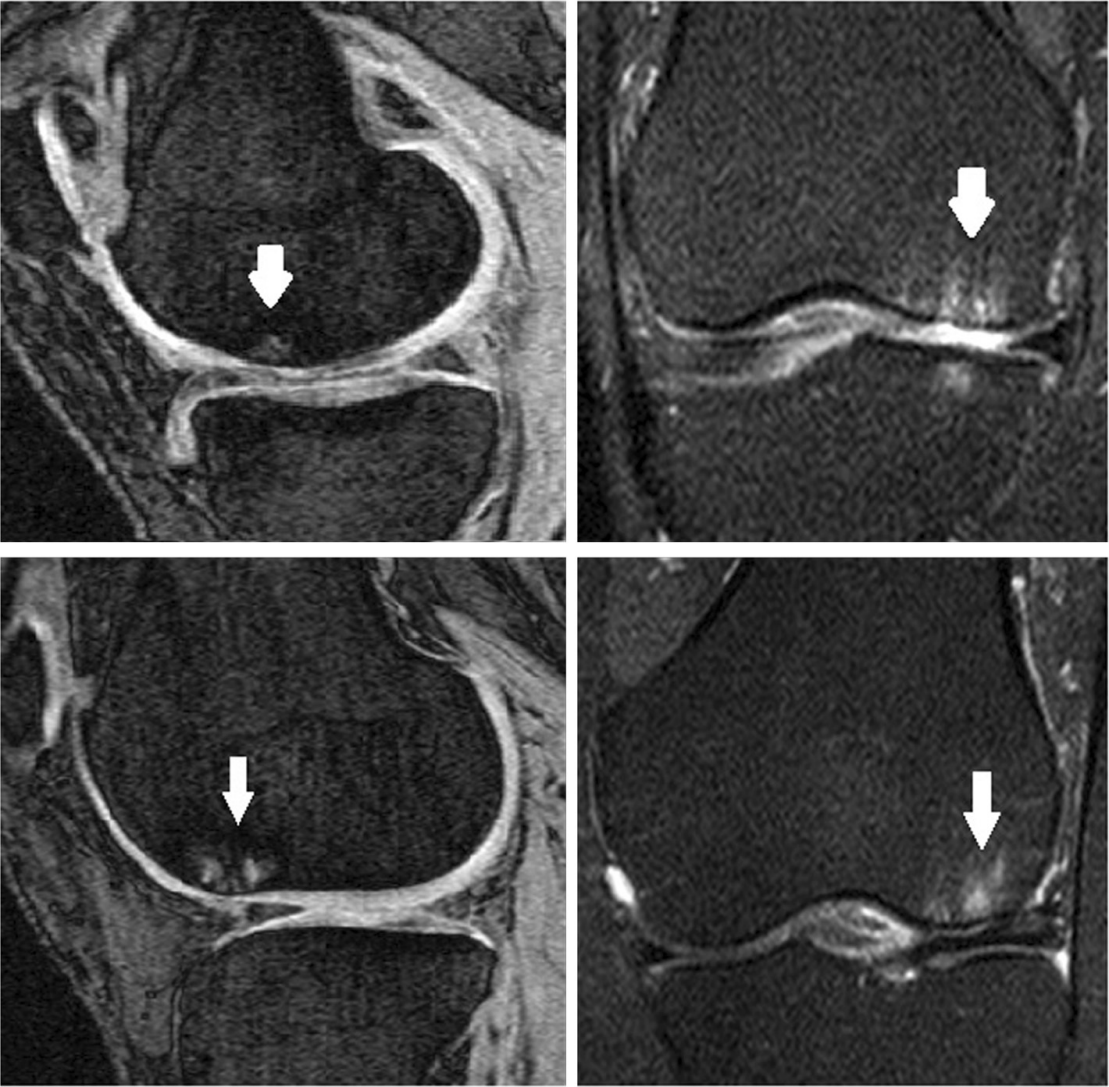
Two BMLs visualised on both T1- and T2-weighted sequences are shown, one on each row. The images on the *left*, obtained in the sagittal plane, show the BMLs identified using T1-weighted sequences. The images on the *right*, obtained in the coronal plane, show the same BMLs imaged using the T2-weighted sequences. *BML* bone marrow lesion
